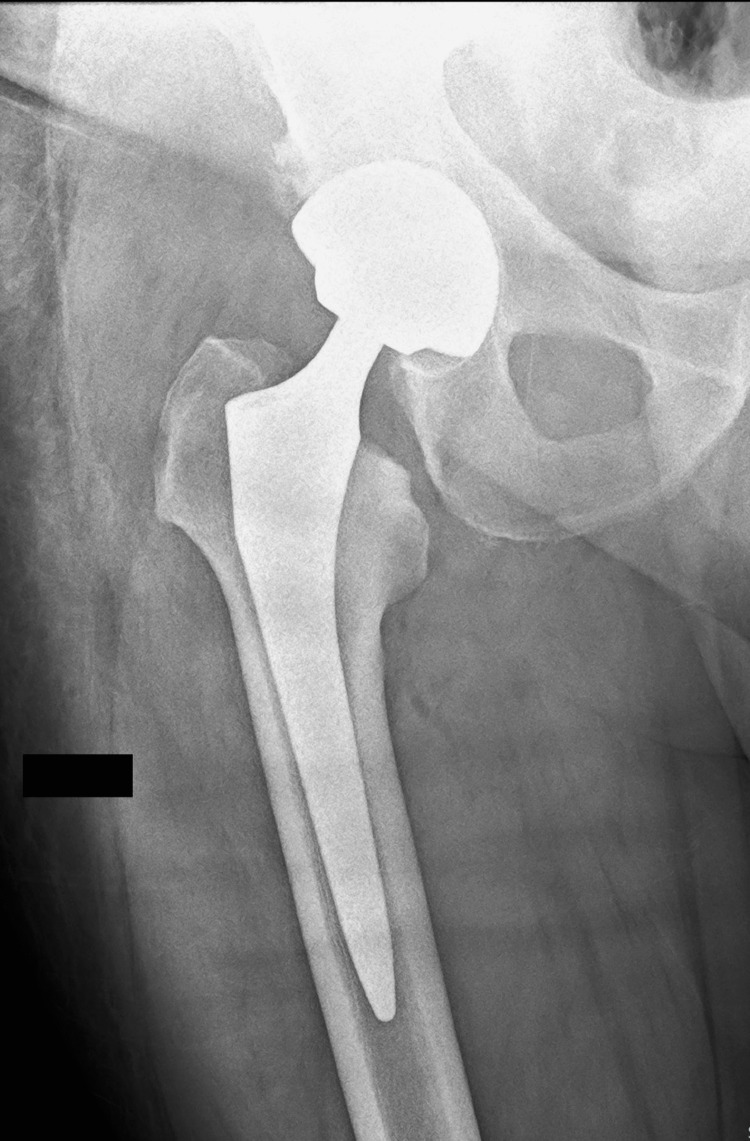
Radiograph of the initial total hip arthroplasty showing the uncemented prosthesis in place after surgery.
